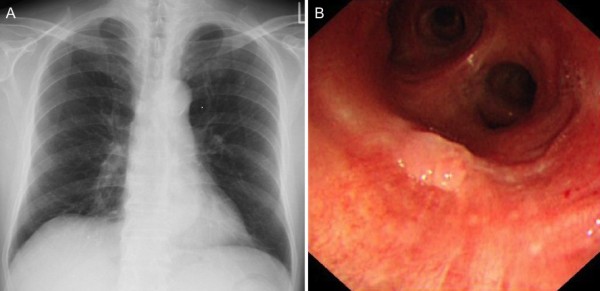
Preoperative examination. a, Chest X-ray showed no abnormalities. b, an elevated lesion was observed in the membranous portion of the left main bronchus, and was diagnosed as squamous cell carcinoma by bronchoscopic biopsy.
